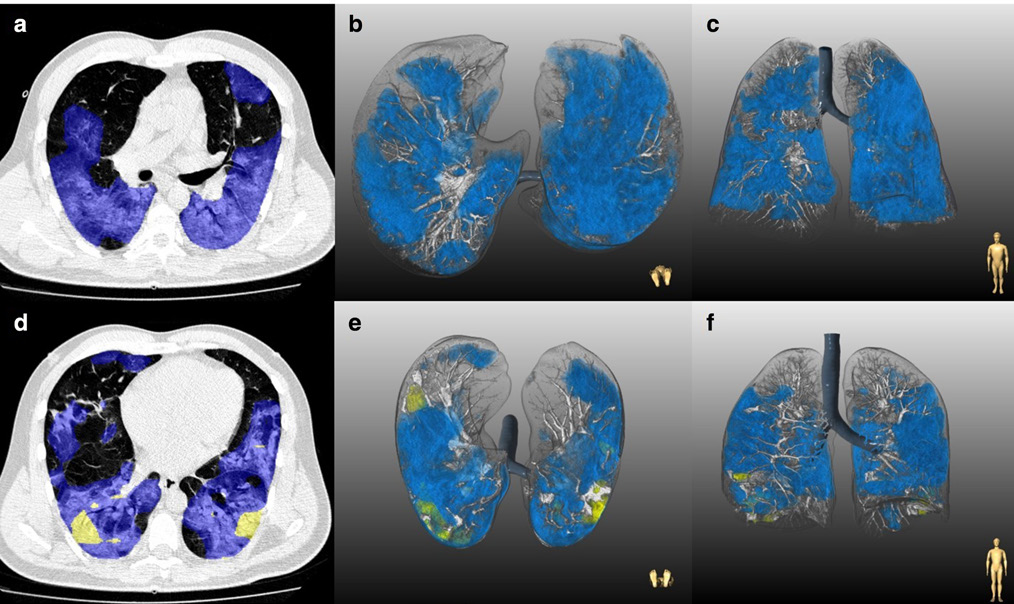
Representative examples of chest-CT findings in patients with non-B.1.1.7 (A–C) and B.1.1.7 (D–F) infection. A–C: Chest-CT study of a 28-year-old male with non-B.1.1.7 pneumonia. The patient had obesity, hypertension and diabetes mellitus. At the time of hospital admission, oxygen saturation was 88% and patient had fever, fatigue, dyspnea, loss of smell and taste, nausea and vomiting. Patient was discharged after an uncomplicated 10-day hospital admission. A: Axial slice depicts bilateral GGOs (blue). Lesion quantification revealed a GGO burden of 42.8% without consolidation. B: Three-dimensional lung renderings in axial and C: coronal planes. D–F: Chest-CT study of a 45-year-old male with B.1.1.7 pneumonia. The patient had normal body mass index and no comorbidities. At the time of hospital admission oxygen saturation was 86% and patient had fever, cough and dyspnea. Patient needed admission to intensive care unit with mechanical ventilation, vasopressor therapy, extracorporeal membrane oxygenation and died 14 days after hospital admission. A: Axial slice demonstrating 40.4% total lesion burden with 39.2% GGO burden (blue) and 1.2% consolidation burden (yellow) at the time of hospital admission. B: Three-dimensional lung renderings in axial and C: coronal planes. GGO, ground-glass opacity.
